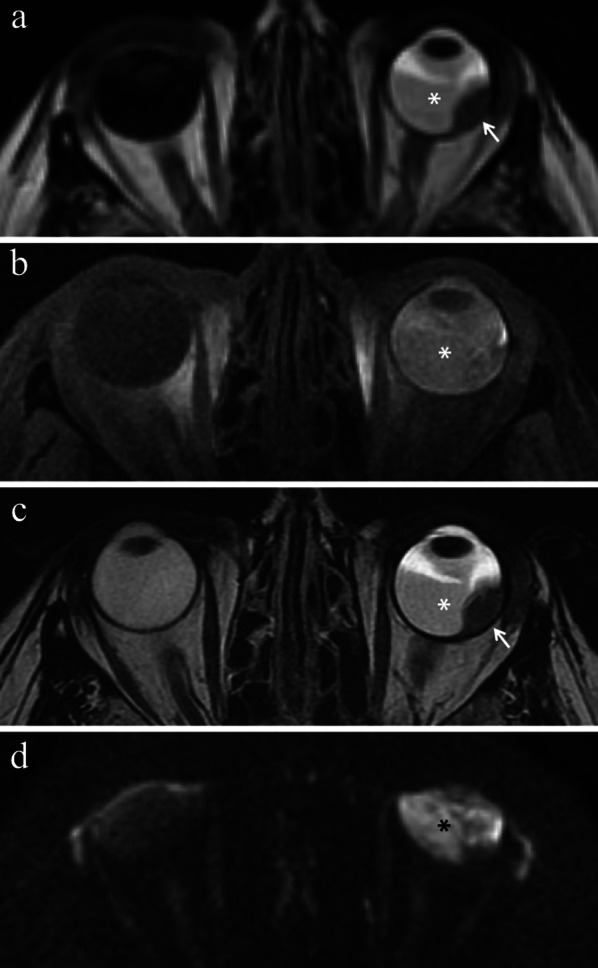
A 75-year-old woman with radiation-induced vitreous hemorrhage after proton-beam radiotherapy for choroidal melanoma of the left eye. The patient underwent secondary enucleation one year after radiotherapy because of painful eye. Axial (a) T2-weighted FLAIR (from brain MRI scan), b fat-suppressed T1-weighted, c T2-weighted turbo spin-echo and (d) DW (b = 1000 s/mm^2^) images. The anterior chamber and the vitreous body of the left eye demonstrate inhomogeneous high signal intensity on T2-weighted FLAIR and T1-weighted images (white asterisks). On T2-weighted image, an intraocular fluid–fluid level, with relative hypointensity of the declivous portion (white asterisk), is recognizable within the left globe. Note the difference with the physiological water-like signal intensity of the contralateral eye. On DW image, the left vitreous body exhibits restricted diffusion with high signal intensity (black asterisk). The findings are consistent with extensive vitreous hemorrhage. A dome-shaped intraocular lesion (white arrows in a and c) is recognizable along the lateral aspect of the left globe
